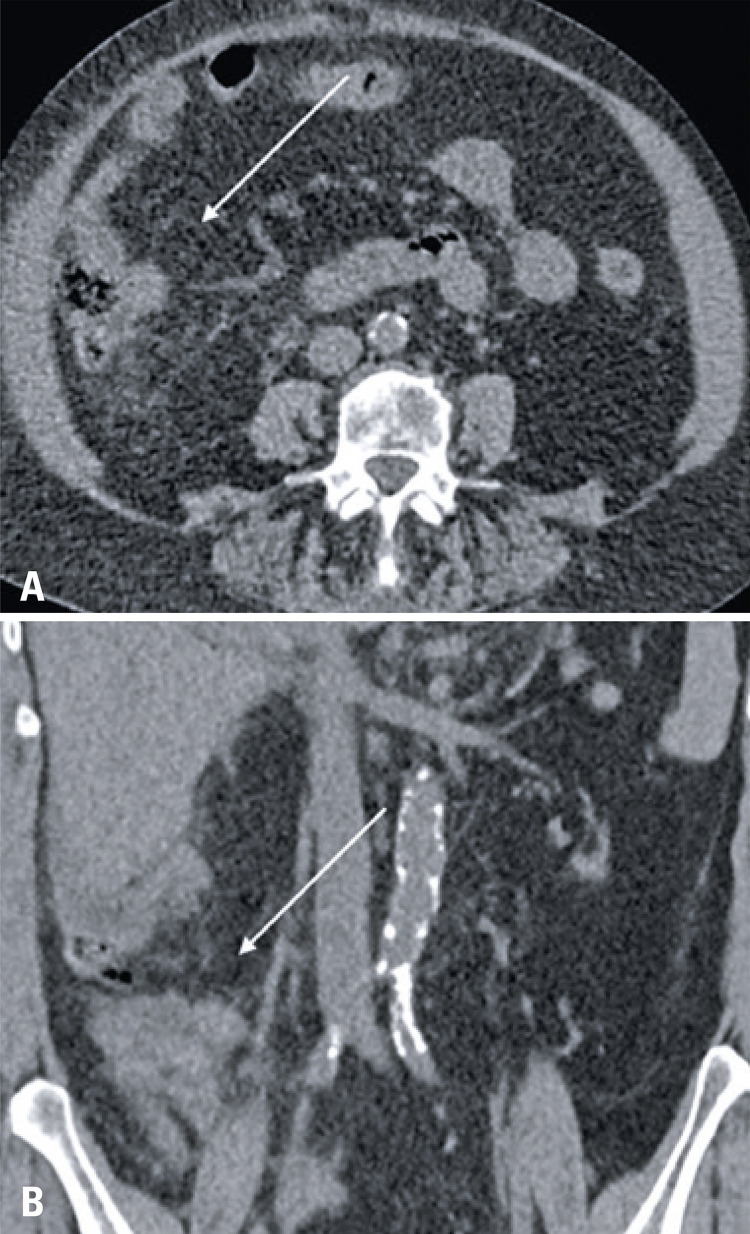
Axial (A) and coronal (B) images of a non-enhanced abdomen computed tomography show thickened walls in the cecum and terminal ileum, with fat stranding (arrows), consistent with enterocolitis
